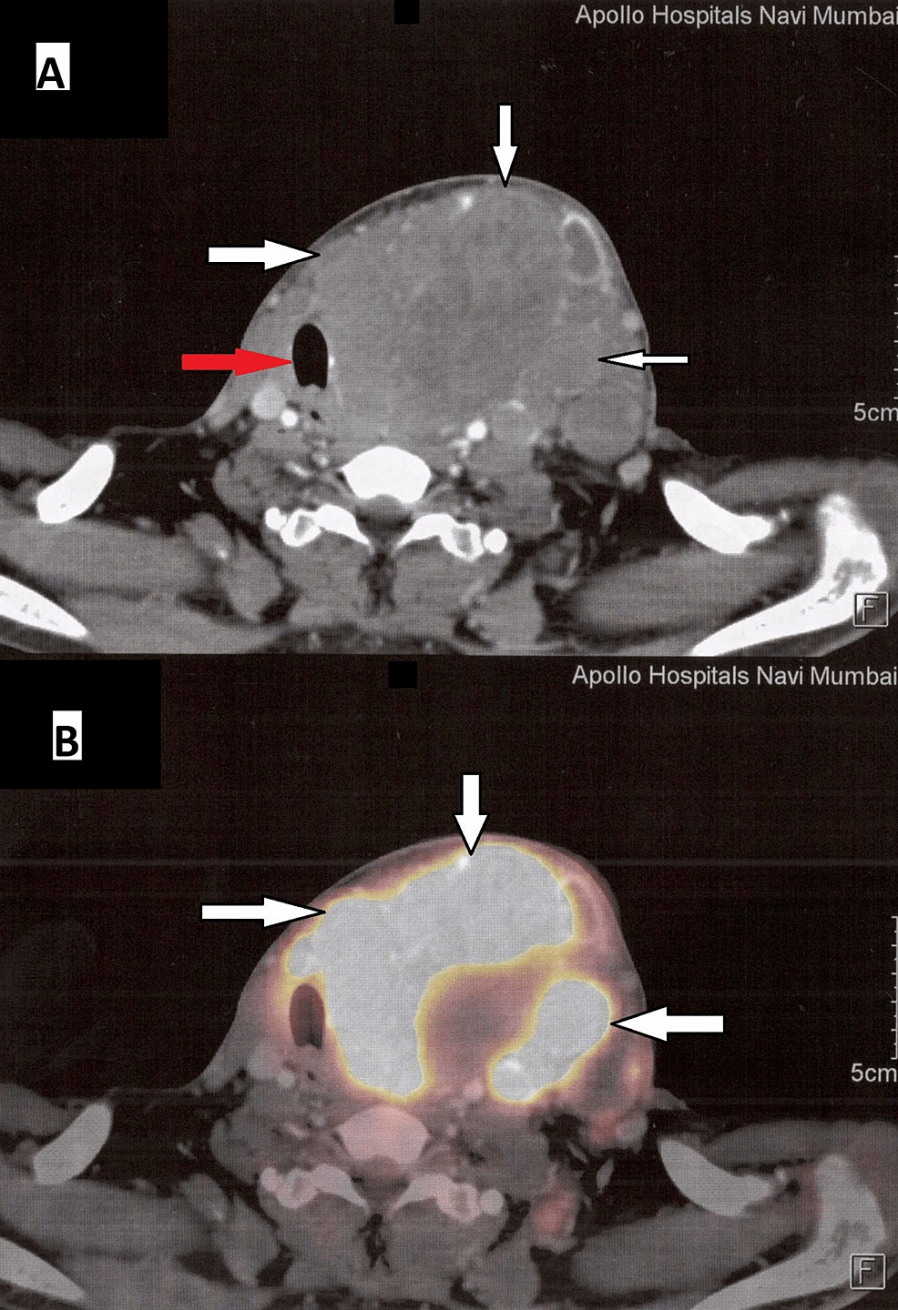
Positron Emission Tomography-Computed Tomography (PET-CT) scan showing soft tissue swelling around the neck. A) Computed Tomography scan showing soft tissue density around the neck involving the left lobe of the thyroid gland (white arrow). The mass compresses and displaces the trachea to the contralateral side (Red arrow). B) PET-CT scan showing enhancing soft tissue mass (white arrow) involving the left lobe of the thyroid gland with increased fluorodeoxyglucose (FDG) uptake (Max SUV:77.11). It also infiltrates the isthmus and the right lobe.
